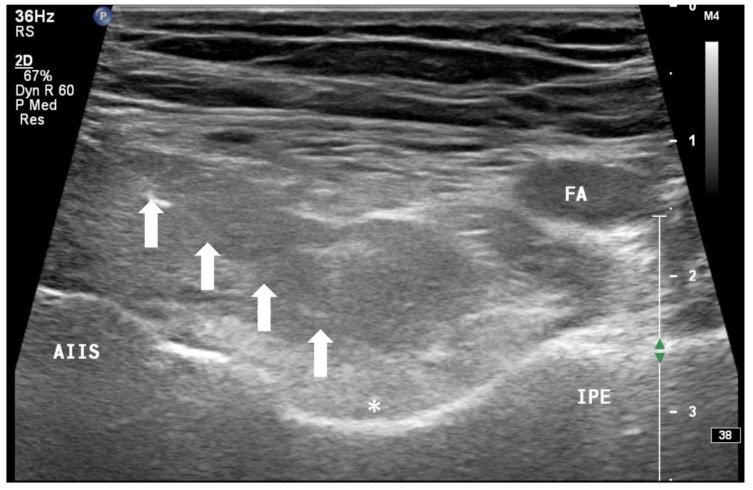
Ultrasound image showing injection of FNAB and AON by infiltration of the drug between the IPE and AIIS from lateral to medial (PENG approach). FA: femoral artery, AIIS: anterior inferior iliac spine, IPE: iliopectineal eminence, *: absolute alcohol spreading underneath iliopsoas muscle above the ASIS-IPE bone surface, white arrow: needle.
